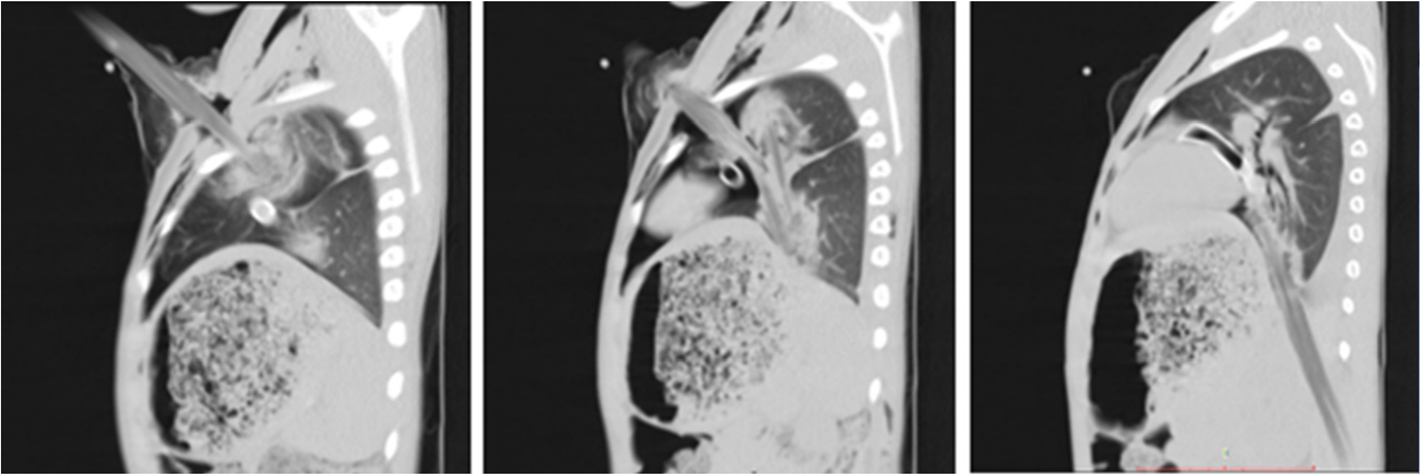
Chest CT showing trajectory of the impalement in the sagittal plane, passing in close proximity to hilum of the lung and the heart. Most inferior extent seems to penetrate the diaphragm
